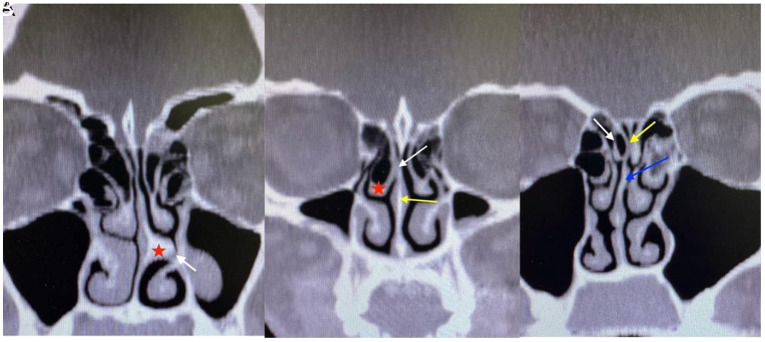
Coronal computed tomography scans show A: septal spur (red star), contact point (white arrow); B: middle concha bullosa (red star), contact point (white arrow), septum (yellow arrow); C: superior concha bullosa (white arrow), contact point (yellow arrow), septum (blue arrow).
